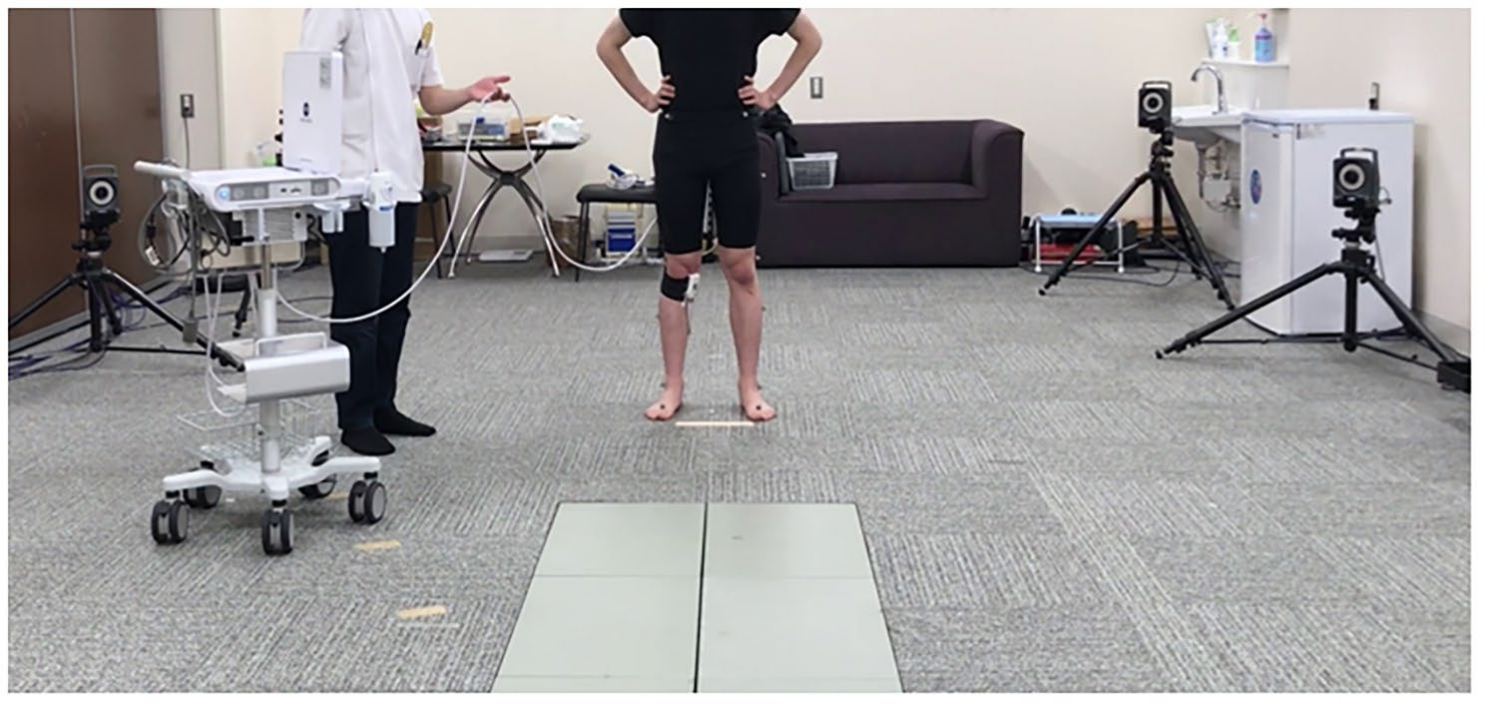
The combination of the ultrasonography and motion analysis systems
